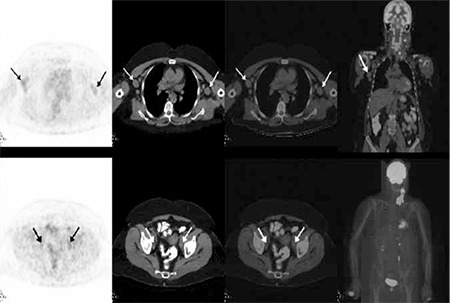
A 64-year-old female patient with CLL/SLL diagnosis. Axial PET, CT, PET/CT fusion and coronal PET/CT fusion images (upper row) of whole-body PET/CT revealed multiple lymph nodes with minimally increased FDG uptake at the right cervical, right supraclavicular, bilateral axillary lymph nodes. There was also minimally increased FDG accumulation at the bilateral internal iliac lypmh nodes (lower row) which was compatible with low FDG uptake of indolent lymphoma.
